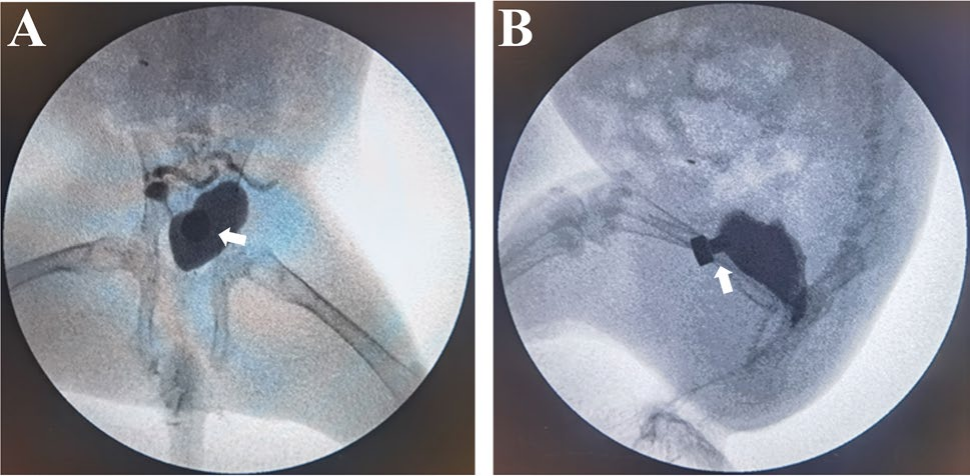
Confirmation of the position of the two magnets by cystography. (A) Anteroposterior radiograph; (B) Lateral radiograph.
